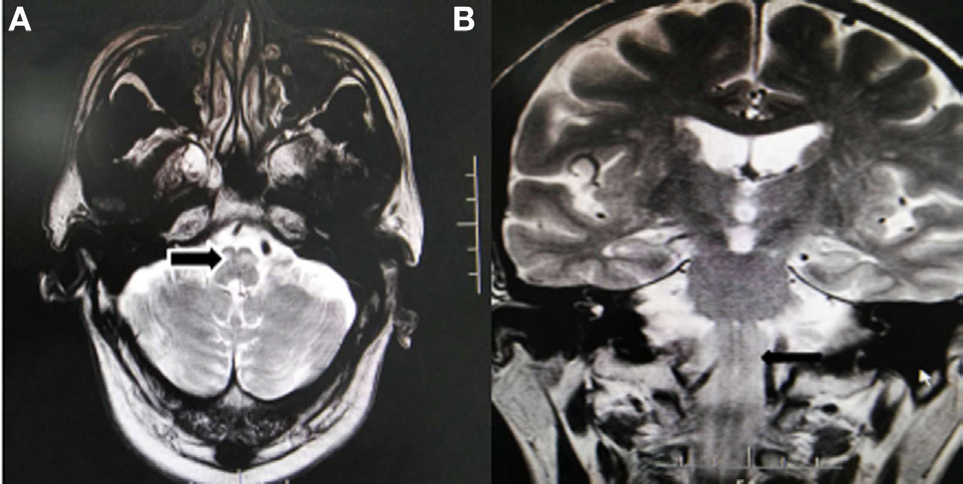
1.5T Brain MRI, axial T2-weighted images (A) and coronal T2-weighted images (B) shows the symmetric enlargement and increased signal intensity of both inferior olives (arrow).
